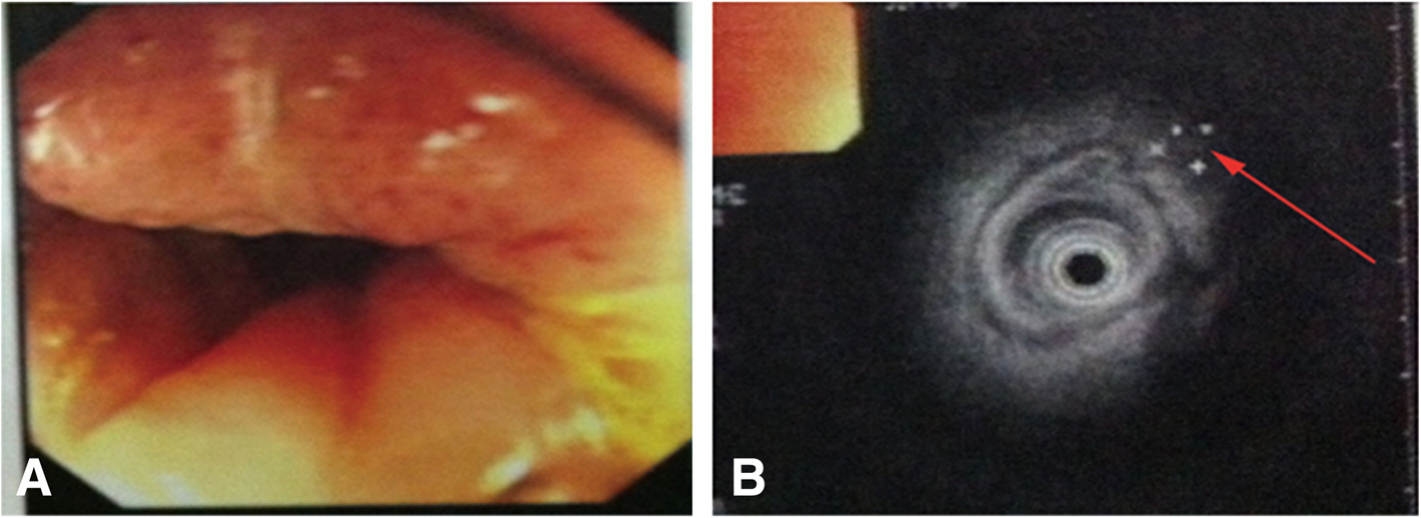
Colonoscopy and rectal endoscopic ultrasound: rectal tumor infiltrating the muscularispropria (T2N0). A: Image under colonoscopy; B: Rectal endoscopic ultrasound.
